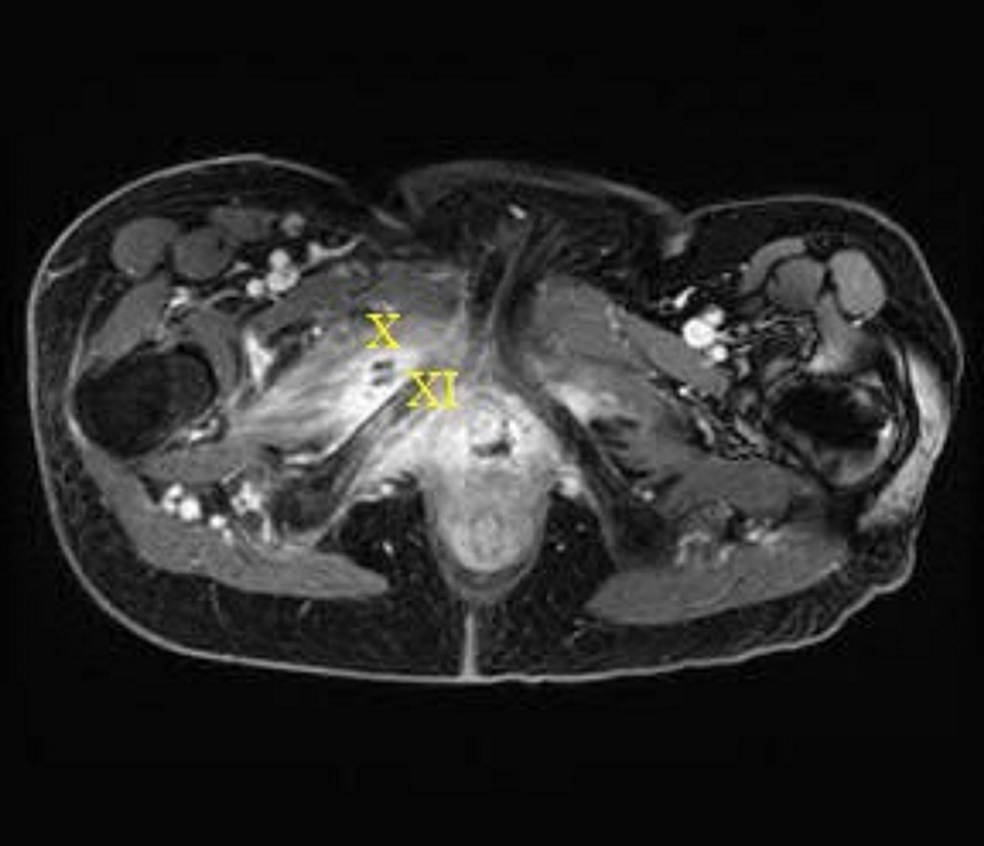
Preoperative MRI, October 2019. Persistent vaginal cutaneous fistula with non-healing right upper thigh wound/exit point X = fistula tract; XI = inflammation and edema (osteomyelitis) of the pubic ramus
